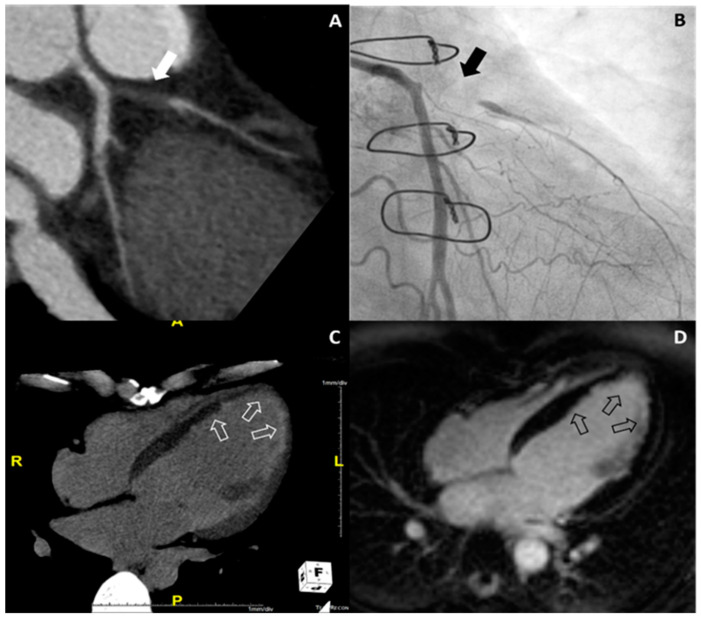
Representative case of ischemic heart failure. On coronary CT angiography (A), total occlusion of the proximal left anterior descending artery (white arrow) is noted, and this finding was confirmed on conventional coronary angiography (black arrow) (B). On delayed-enhanced dual-energy CT with the four-chamber plane (C), subendocardial delayed enhancement is noted on the apical left ventricle and septal wall of the mid left ventricle (white open arrows), which was confirmed on cardiac MR (black open arrows) (D).
